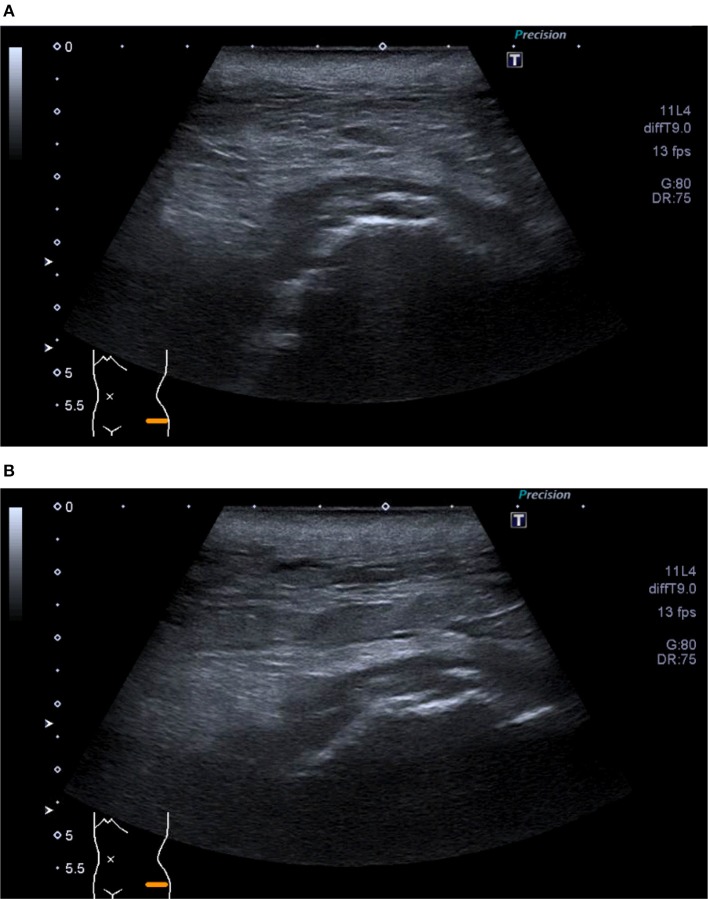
(A,B) Ultrasound of left hip—Superficial collection at site of wound tracking down to deep hip joint surface. Extension to borders of skin markers.
